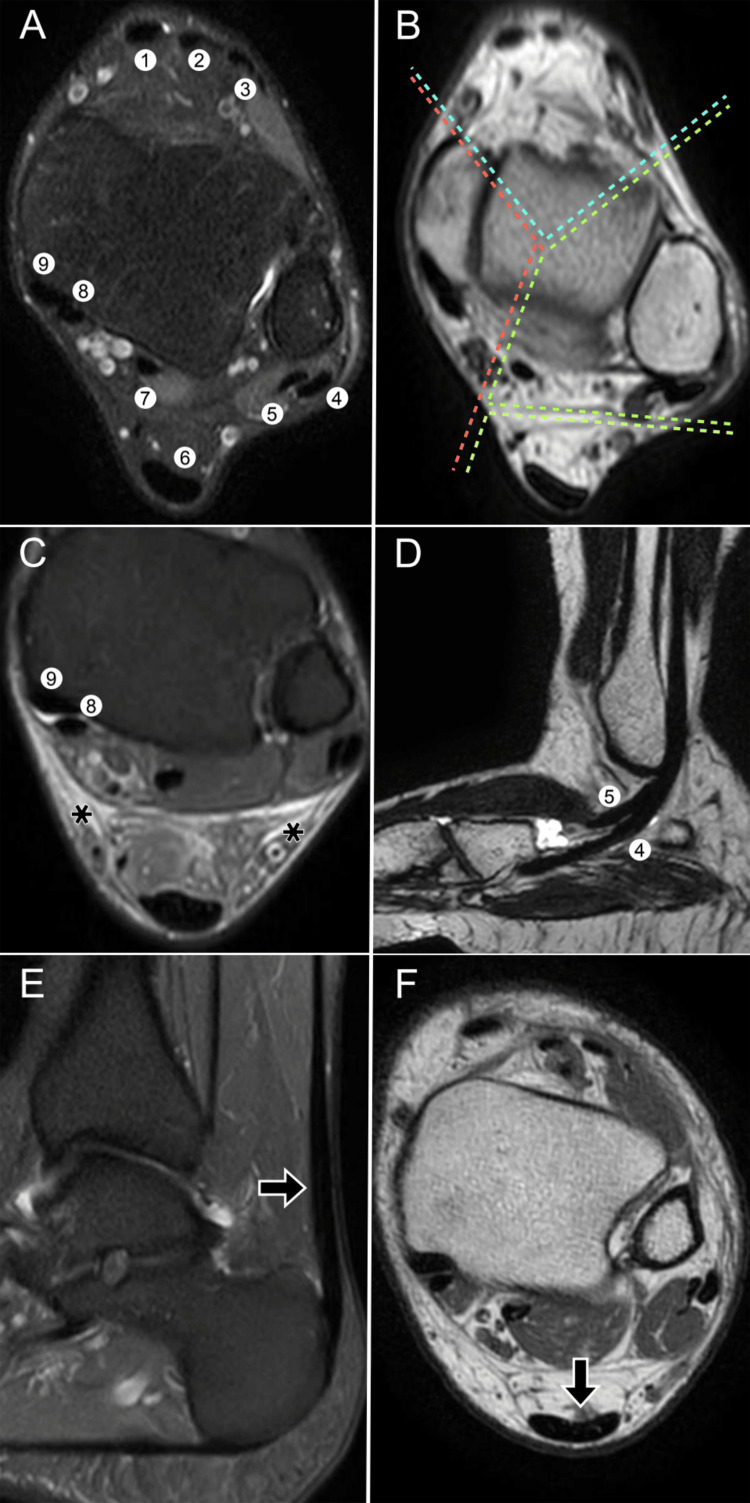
Tendinous compartments of the ankle on MRI. (A) Axial DPFS MRI (dual proton fat-saturated sequence) at the tibio-talar syndesmosis shows (1) tibialis anterior, (2) extensor hallucis longus, (3) extensor digitorum longus, (4) peroneus longus, (5) peroneus brevis, (6) calcaneal (Achilles) tendon, (7) FHL tendon, (8) flexor digitorum longus, and (9) tibialis posterior. (B) The right panel (axial T2 MRI at the talar dome) divides anterior (blue), medial (orange), lateral (green), and posterior (yellow) compartments. (C-D) Axial DPFS MRI shows the posterior tibial (9) and flexor digitorum longus (8) tendons with tenosynovitis (*) and surrounding edema; the right panel shows a multiplanar reconstruction of the peroneus longus (4) and brevis (5). (E-F) Sagittal DPFS MRI depicts the Achilles tendon; axial T2 MRI confirms its normal hypointense signal and semilunar morphology (arrow). Images belong to the authors; patient consent was obtained.
